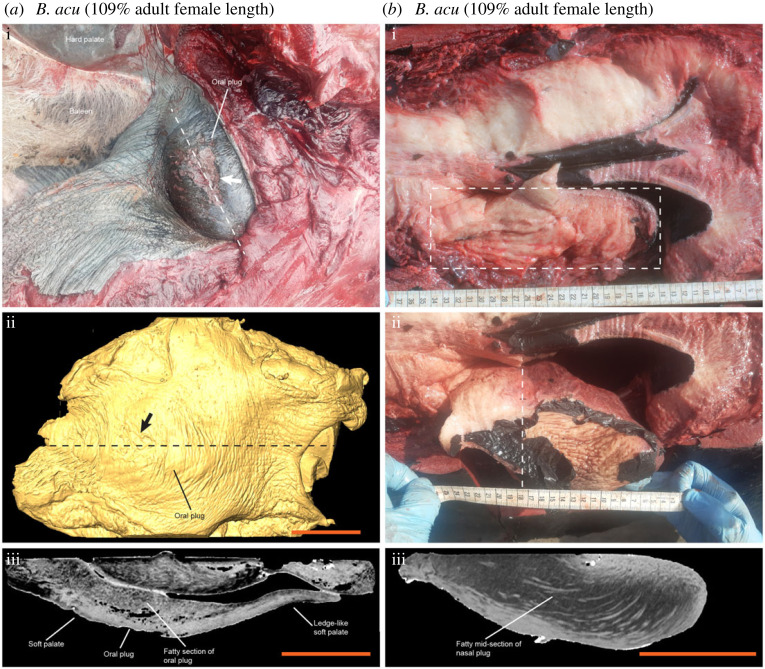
Oral and nasal plugs in adult minke whale. (*a*) On (i), photo of oral plug in adult minke whale (*Balaenoptera acutorostrata*, *B. acu*). A recognizable abrasion mark is labelled with white arrow. This photo from the beached minke whale lying on its back has been rotated so the hard palate appears at the top of the image as it would if the whale was approximately right-side up. In (ii), X-ray computed tomography surface reconstruction of excised oral plug with abrasion mark labelled with a black arrow. At (iii), sagittal X-ray computed tomography slice through the oral plug along the dashed lines in (i) and (iii) images. A hypointense section of the soft palate reveals a fatty portion of this structure in a mature rorqual. (*b*) On (i) and in (ii), photos of nasal plugs in place (i) and with the left nasal plug partly resected (ii) in adult minke whale. At (iii), coronal X-ray computed tomography slice through the left nasal plug along the dashed plane and lines in (i) and (ii) images. A hypointense mid-section of the nasal plug reveals at fatty portion of this structure in a mature rorqual. Scale bars represent 100 mm on all images.
